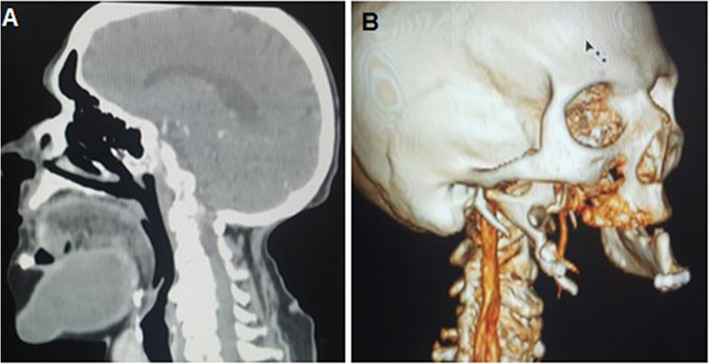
CT scan of the recurrence: Post-contrast sagittal reformatted CT image (a) shows large thick-walled necrotic cystic lesion at the right mandibular and submandibular regions with an erosion of the underlying bone graft. Volume rendering (VR) reconstructed CT image (b) confirms the destruction of the right-sided bone graft by the recurrent mass
